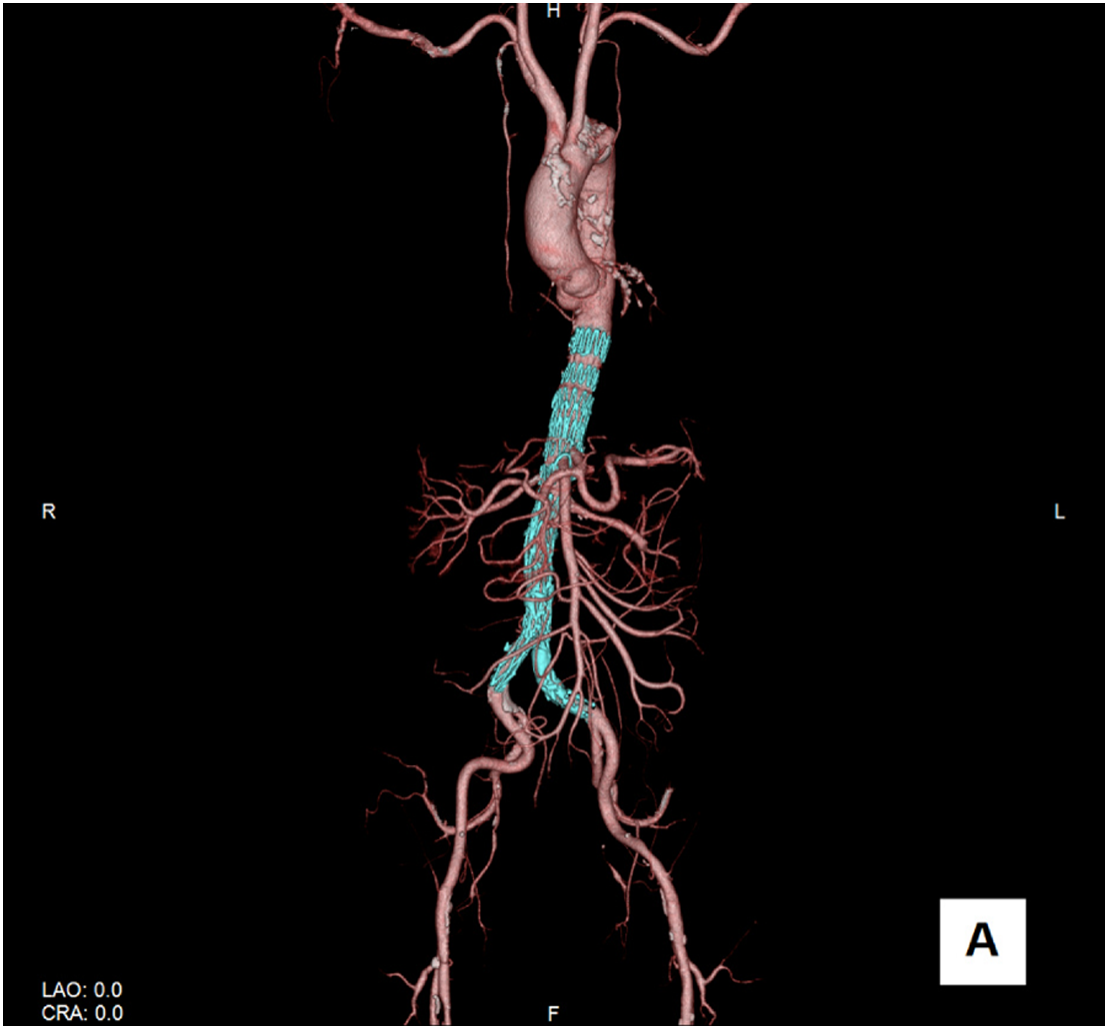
Fig. 3 Postoperative contrast-enhanced computed tomography (CT) examination shows no endoleak and patency of the abdominal branches.
